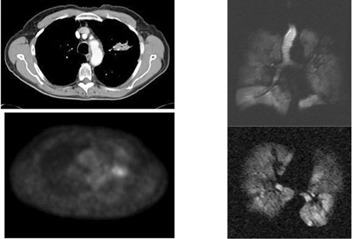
Patient #4 – left lower lobe space occupying lesion seen on CT, PET and MRI.
